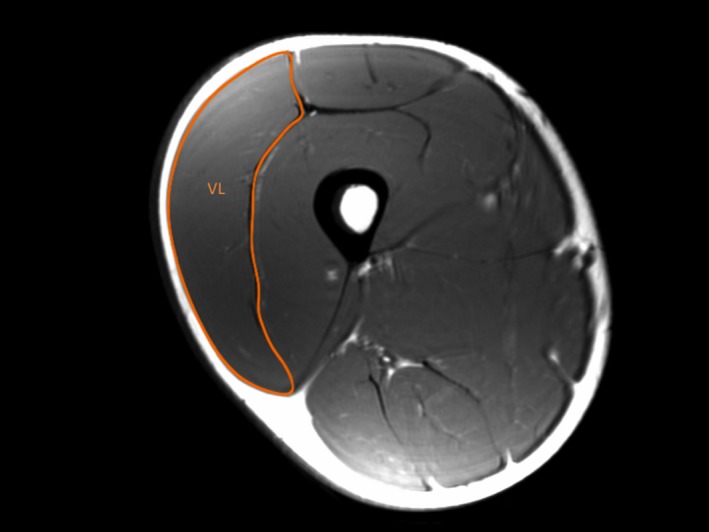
Magnetic resonance image scan of the right thigh at 50% of femur length from a representative subject. The contours that comprise the *vastus lateralis* (VL) anatomical cross‐sectional area are shown
